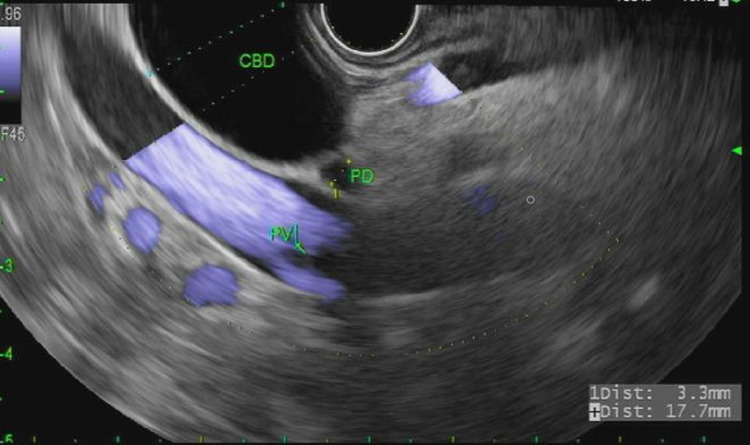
Endoscopic ultrasound showing dilated common bile duct in the head of the pancreas. CBD: common bile duct, PD: pancreatic duct, PV: portal vein
